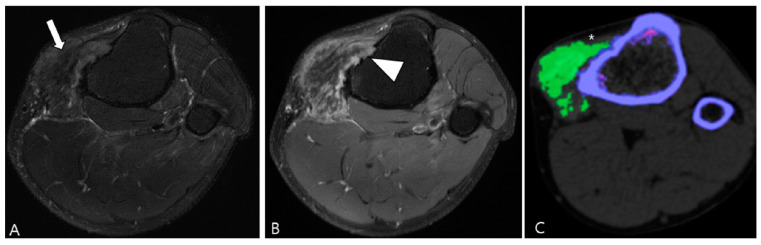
A 47-year-old male with tophaceous gout of the knee. (A) Axial fat-suppressed T2WI reveals a lobulated extra-articular slightly hyperintense soft tissue mass containing low SI foci along the Pes anserine bursa (arrow). (B) Axial contrast-enhanced fat-suppressed T1WI shows heterogeneous enhancement and focal cortical erosion (arrowhead) at the proximal medial tibia. (C) Dual-energy CT reveals the lesion is tophi with MSU deposition of green color coding (asterisk). Trabecular or cancellous bone is displayed in purple.
